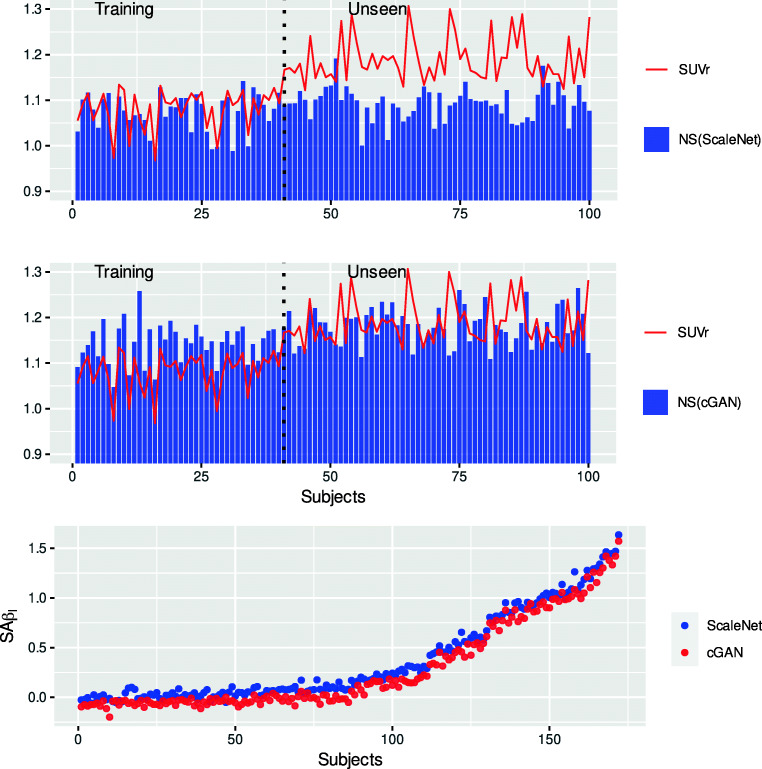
(Top and middle) NS measured in the GM from the ScaleNet and cGAN estimates of 100 subjects (40 training and 60 unseen) with low SUVr (< 1.3). The red line gives the corresponding SUVr values measured from the actual PET scans. (Bottom) Specific activity computed for the whole cohort using the NS estimates generated by ScaleNet and cGAN. Subjects were ordered on the X-axis with increasing SUVr values
